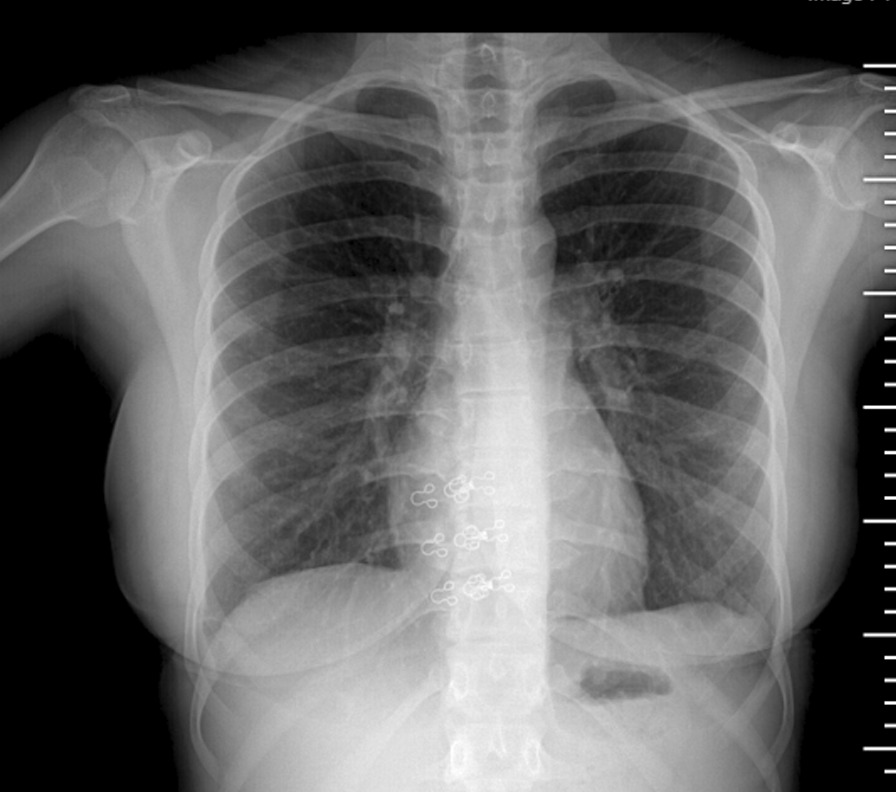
End-of-treatment lung X-ray
